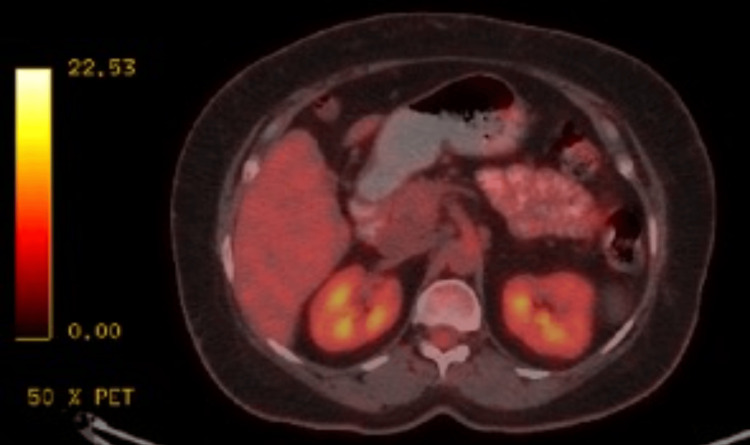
Positron emission tomography/CT showed a complete metabolic response at sites of previous lymphoma involvement.
